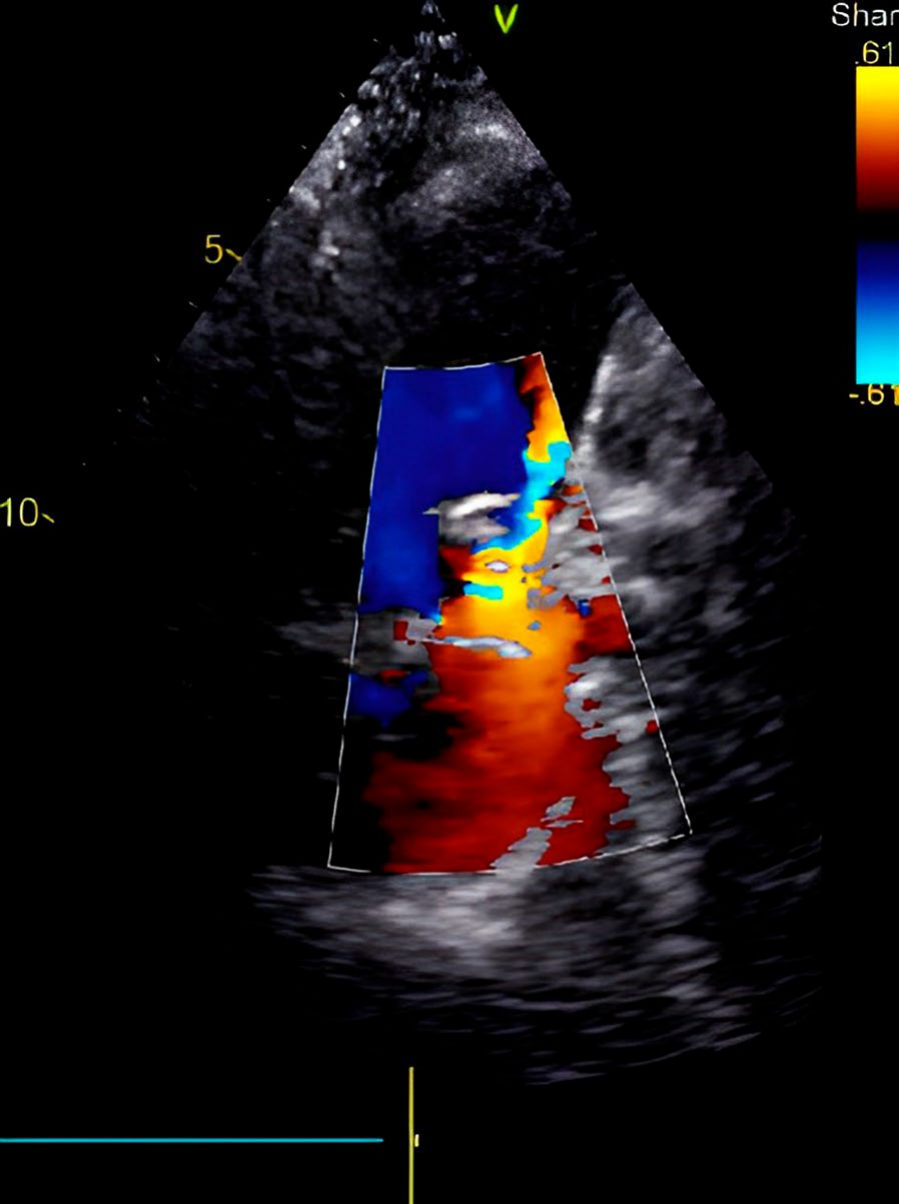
Parasternal short axis view on transthoracic echo showing significant Peri device leak
